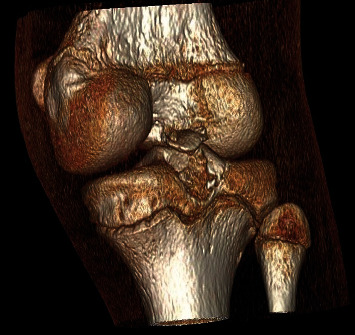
CT scan of Case 1. The 3D reconstruction shows the avulsed tibial fragment from the posterior tibial plateau.
